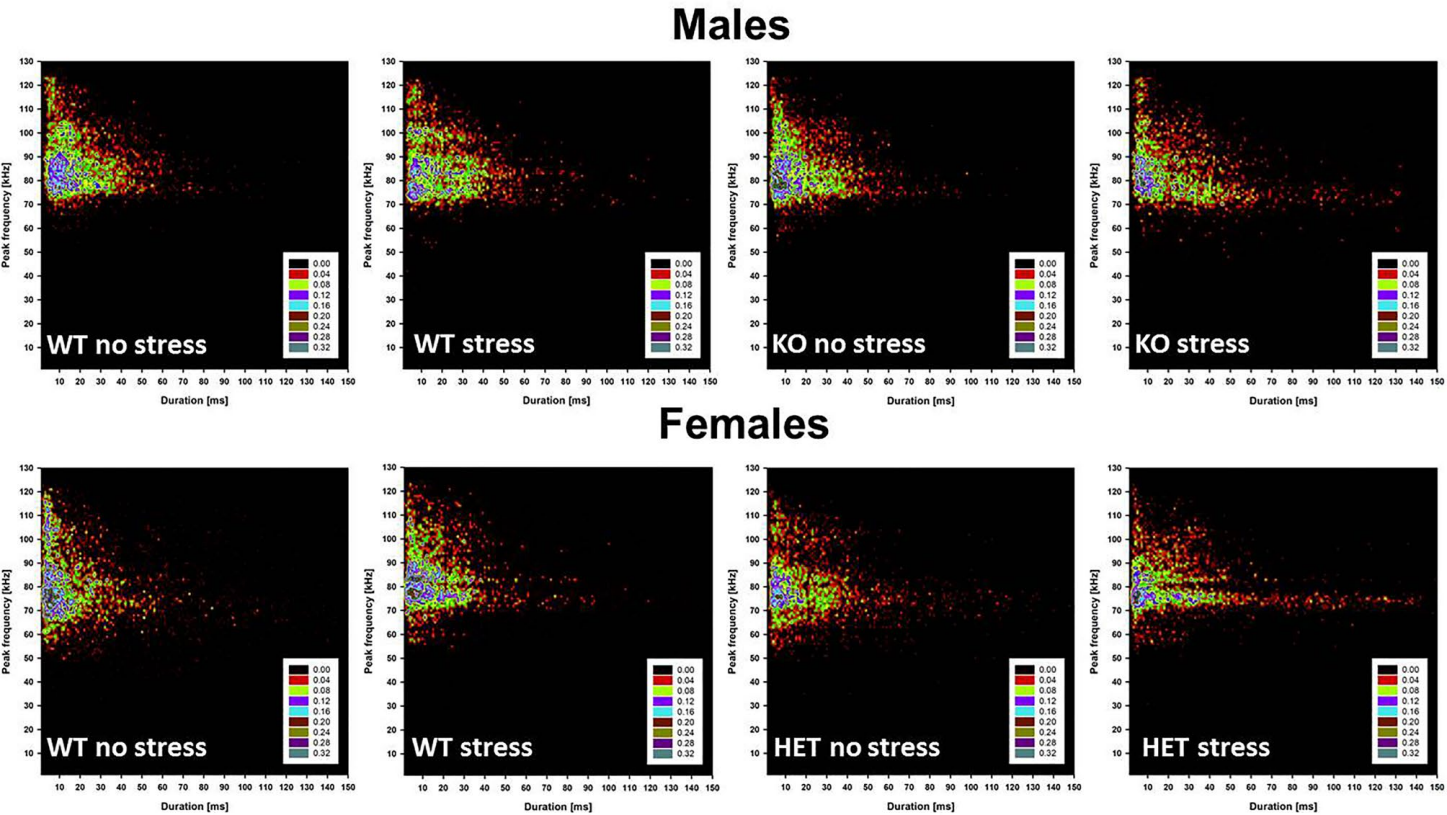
Density plots of individual ultrasonic calls. Density plots depict the distribution of individual USV emitted during 3-min social interaction with a NMRI adult stimulus female, plotted by frequency in kHz and duration in ms. Color coding reflects frequency in percentages.
